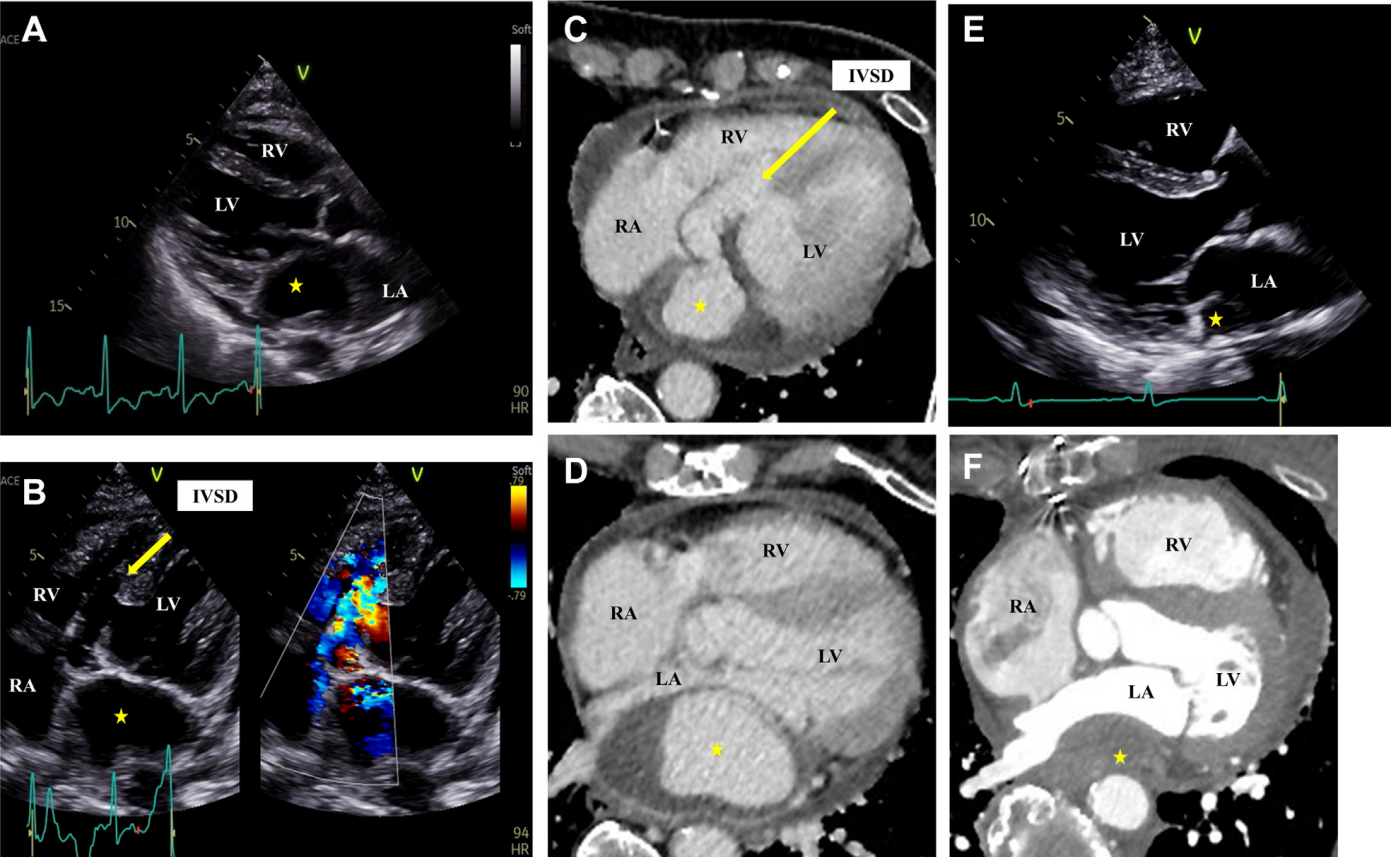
Imaging of the Interventricular Septal Dissection and Pseudoaneurysm Before and After Operation (A, E) Transthoracic echocardiogram, left sternal border approach and long-axis view. (A) The pseudoaneurysm (yellow star) compressed the left atrium (Video 1). (B) Transthoracic echocardiogram, apical 4-chamber view highlighting the IVSD as well as the RA, RV, LA, and LV (Video 2). (C, D, F) Contrast-enhanced computed tomography axial view. (C) IVSD extending to the left atrium. (D) The left atrial outflow tract is significantly compressed by the pseudoaneurysm. (E, F) Four months after surgery, the pseudoaneurysm was regressed (yellow star). IVSD = interventricular septal dissection; LA = left atrium; LV = left ventricle; RA = right atrium; RV = right ventricle.
